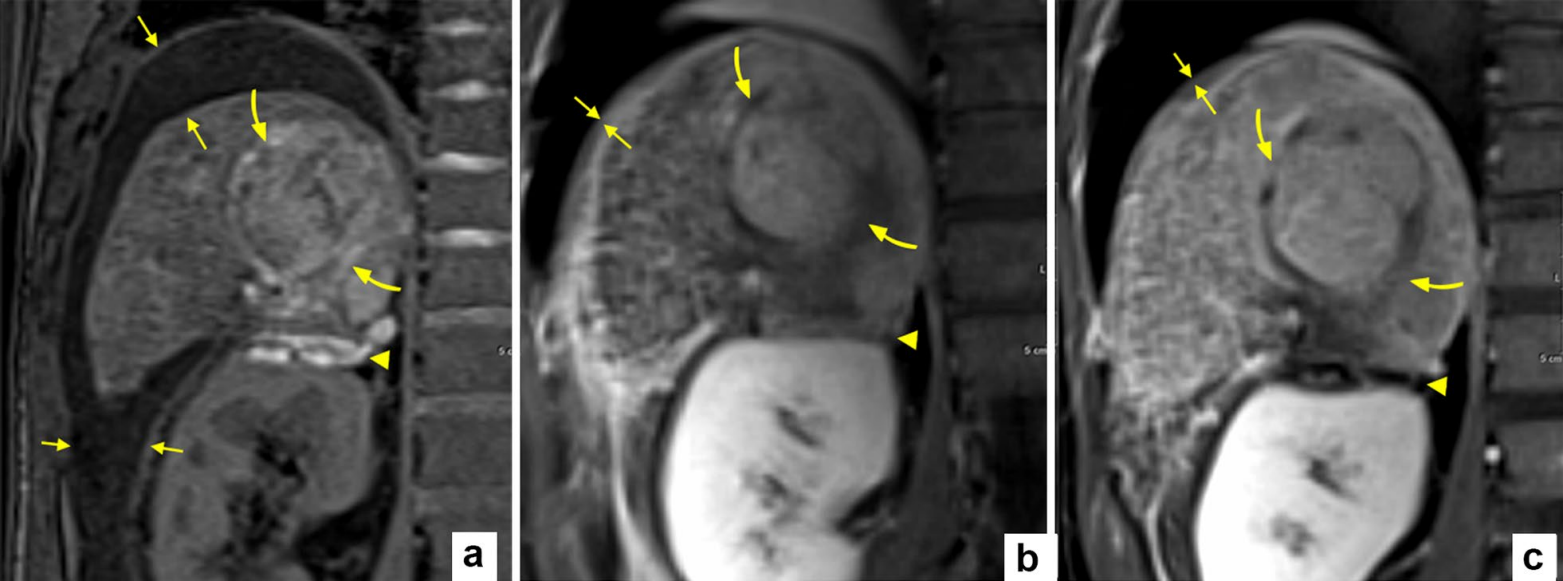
The follow-up magnetic resonance images (MRI). The coronal MRI at 1 (a), 6 (b), and 9 months (c) after HCl-enhanced RFA show reduced lesion size and a satisfactory tumor capsule beyond the hepatic contour (*curved arrow*). The gradually distinct margin of the upper renal fascia is also visible (*arrowhead*). Perihepatic and perirenal ascites are decreased (*twin arrows*)
